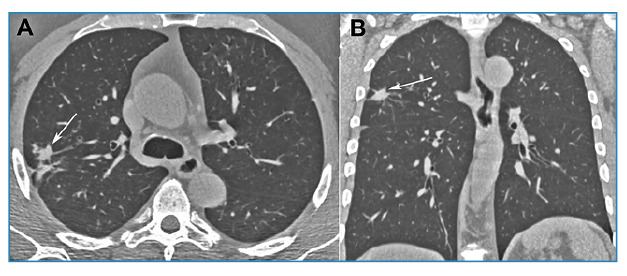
Control chest CT images with axial (A) and coronal (B) reconstruction, obtained 1 year after the start of treatment, show only residual fibrotic lesions (arrows).
